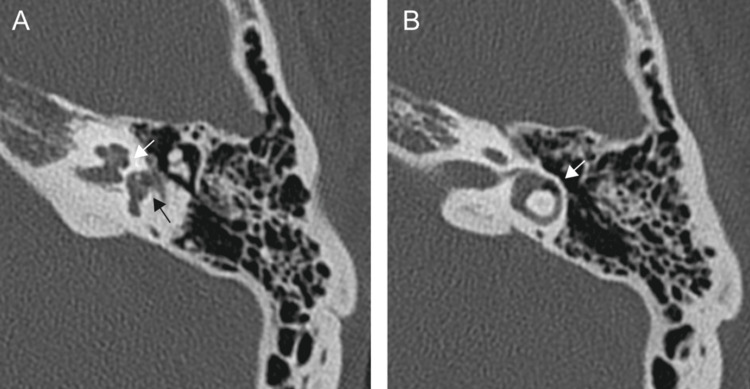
Preoperative CT scans. (A) The black arrow points to the vestibule in which air bubbles and depressed stapes are seen. The white arrow points to the basal turn of the cochlea in which air bubbles are seen. (B) The arrow points to the lateral semicircular canal in which air bubbles are seen.
